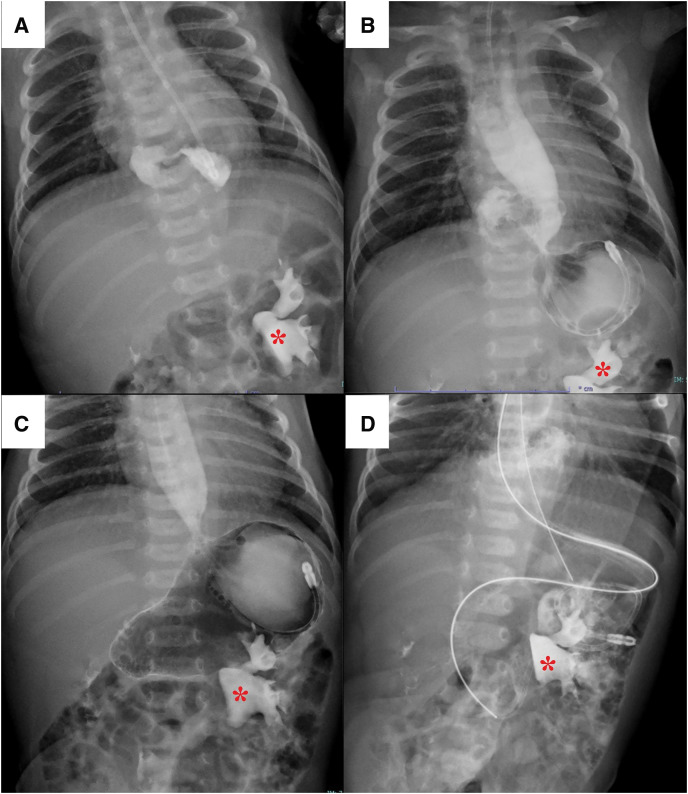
Upper gastrointestinal contrast study. Left renal pelvis distended due to hydronephrosis: contrast medium remained after the contrast CT (*). (A) The abdominal esophagus and cardia were compressed and bent, causing the contrast material to stagnate. (B) When air was insufflated into the stomach, the stomach in the abdominal cavity was observed. (C) As the stomach expanded, the prolapsed portion returned to the abdominal cavity. After hernia reduction, the stomach had a normal morphology. (D) A 5 Fr. enteral tube was placed at the level of the jejunum beyond the ligament of Treitz.
